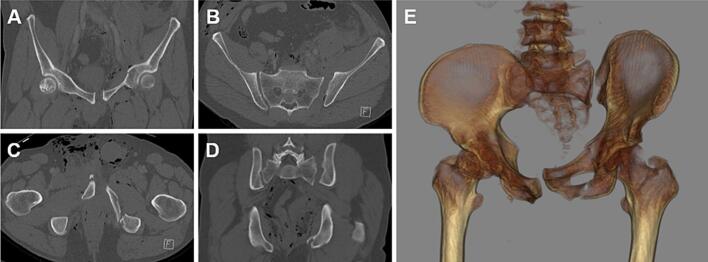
CT scan of the initial pelvic fracture (AO61C2.2) with instability of the symphysis (A, C) and bilateral instability of the posterior pelvic ring in coronal and axial plane (B, D). 3D reconstruction of the initial CT scan (E).
